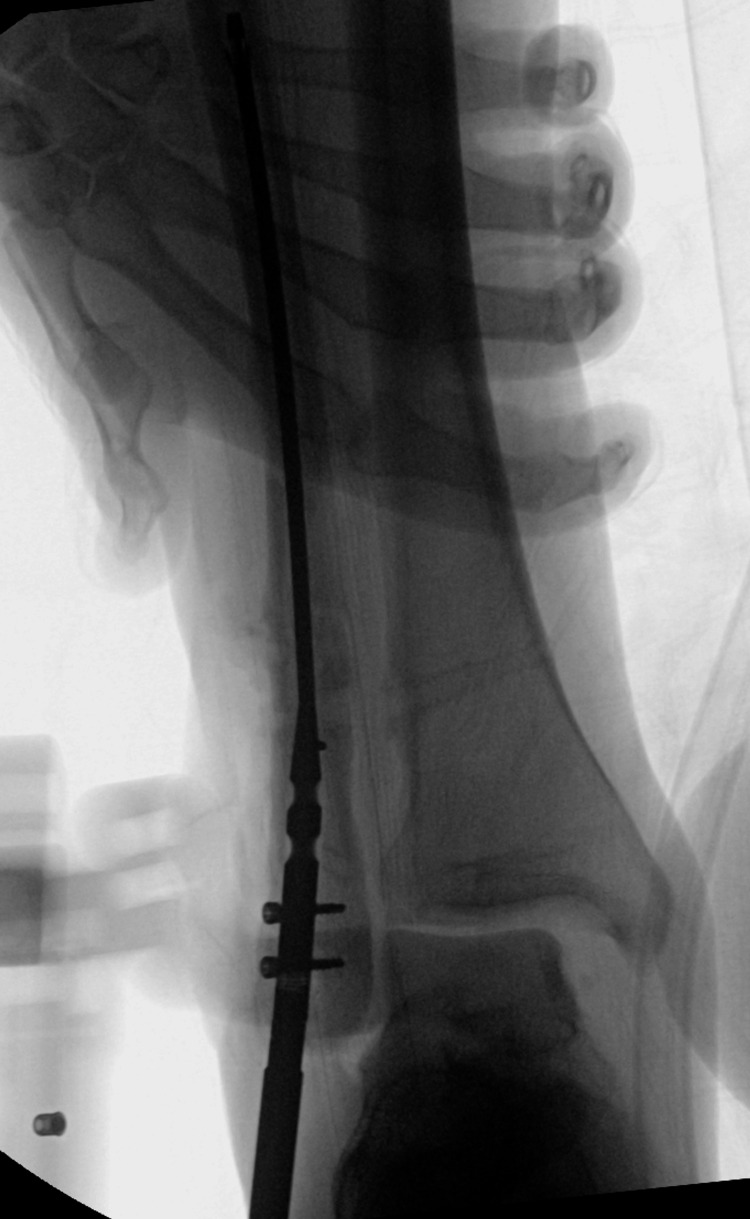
Intraoperative radiograph performing an external stress depicting medial clear space widening as well as talar lateral shift indicative of syndesmotic instability
